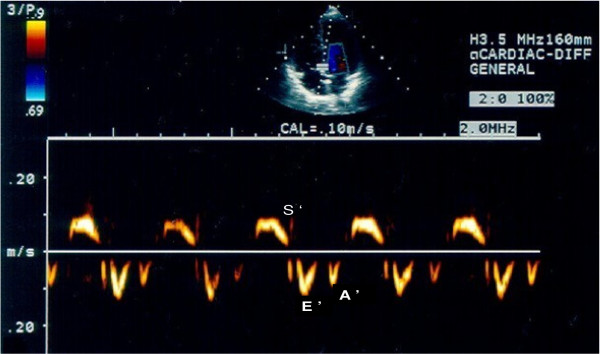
LV diastolic pattern by tissue Doppler. S': LV systolic myocardial displacement velocity; E': LV early diastolic displacement velocity; A: LV late diastolic displacement velocity.
